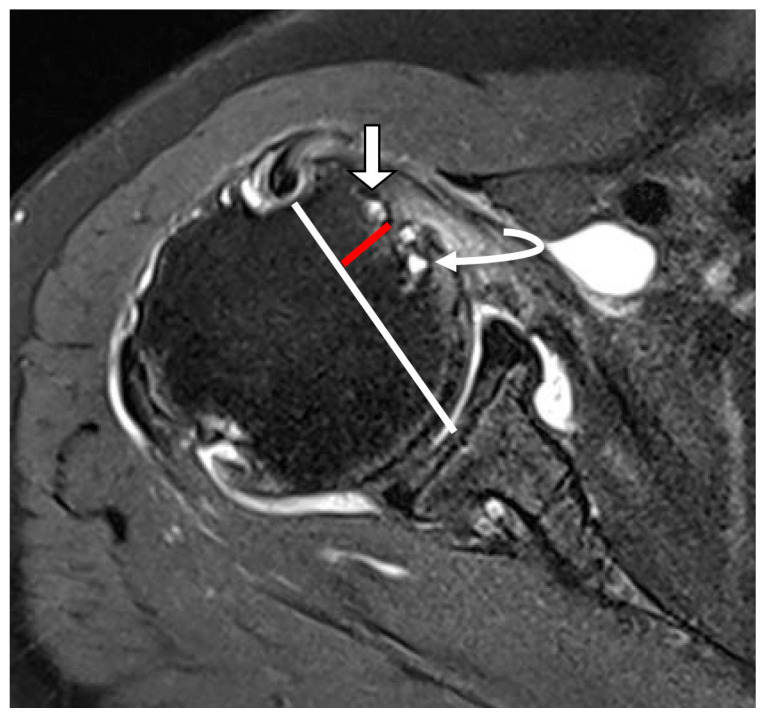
Cystic lesion in the lesser tuberosity on T2-weighted oblique axial fat-suppressed image. A white line extends from the bicipital groove to the midpoint of the glenoid fossa at the level of the lesser tuberosity. A red line indicates the border of a bony protuberance called the lesser tuberosity. Cysts located within the bony protuberance were defined as cysts within the lesser tuberosity (straight arrow). Cysts adjacent to the bony protuberance were defined as cysts adjacent to the lesser tuberosity (curved arrow).
